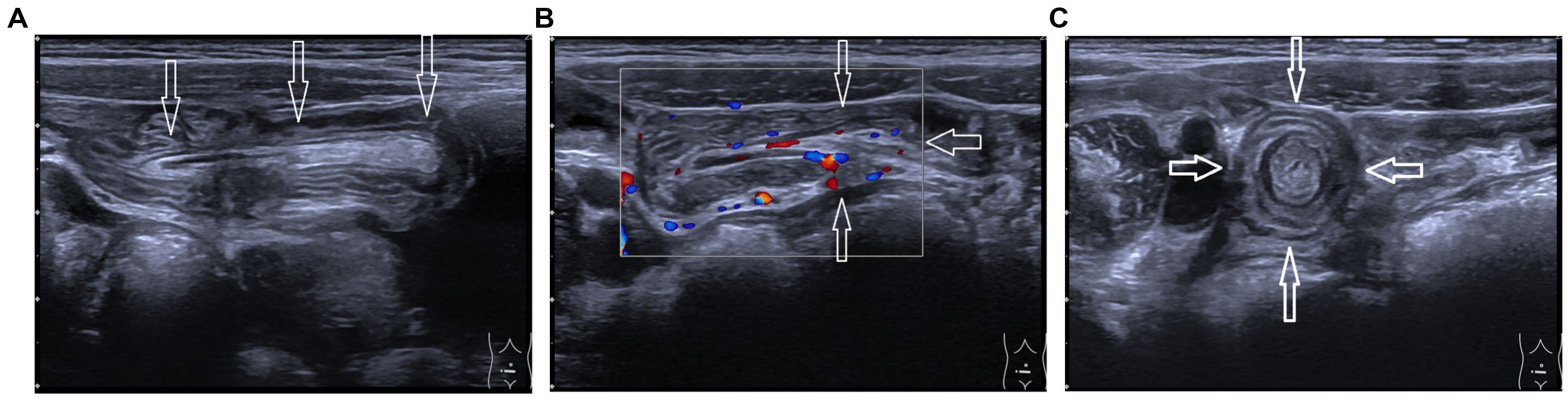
High-frequency ultrasonographic images of inverted Meckel’s diverticulum. (A) White arrow indicates the “glove-finger”-shaped protrusion into the ileal lumen. (B) The white arrow shows the hyperechoic mesentery and tubular anechoic mesenteric vessels extending outward and continuing with the adjacent mesentery. (C) Transverse section displays the “target sign” with a hyperechoic center (arrow).
